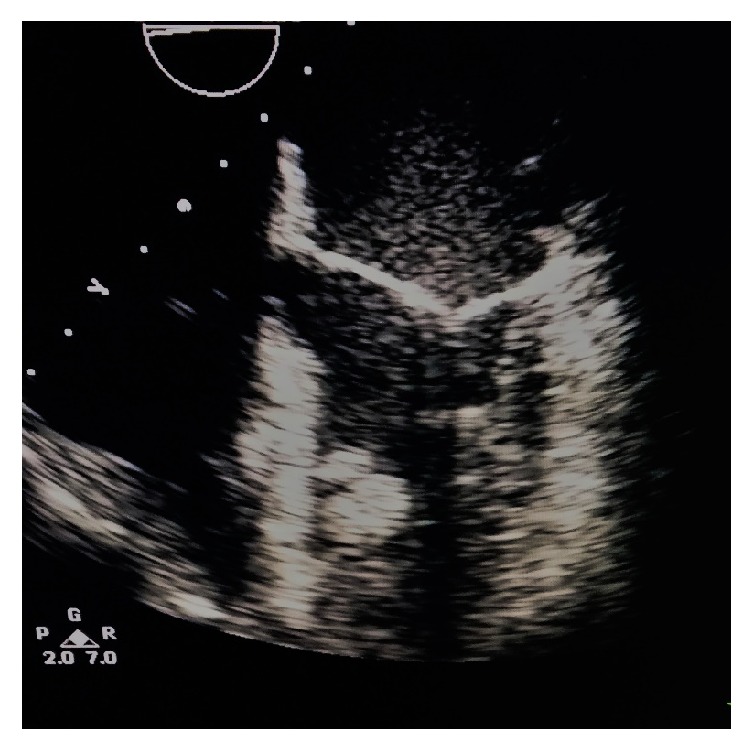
Transesophageal echocardiography showed two pedunculated thrombi in the left ventricle.
